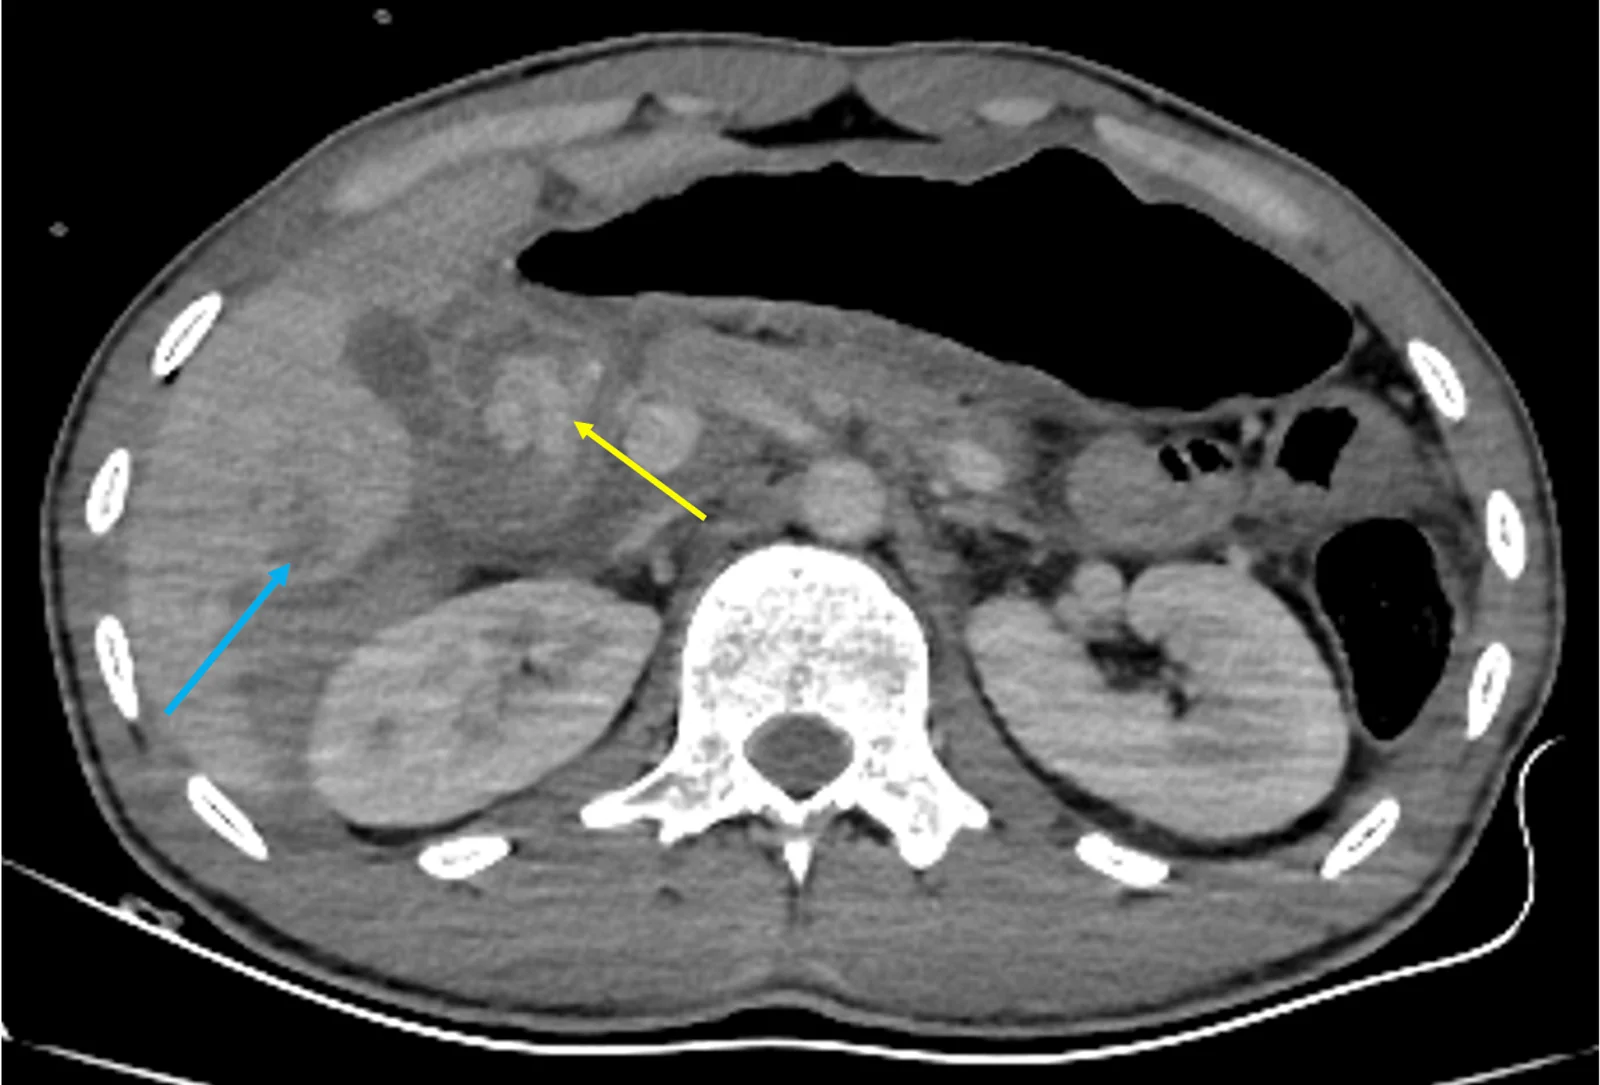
Imaging findings from abdominal post-contrast CT (portal phase) Axial abdominal CT post IV contrast at portal phase shows contrast endoluminal extravasation (yellow arrow), and segment VI hepatic laceration.
